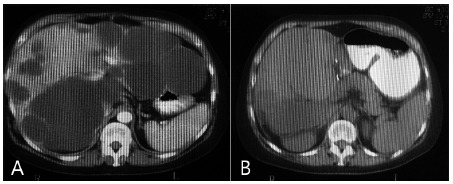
A) CT-Scan shows grade II APLD compromising left later segment and posterior segments of the right lobe; B) post-operative result
